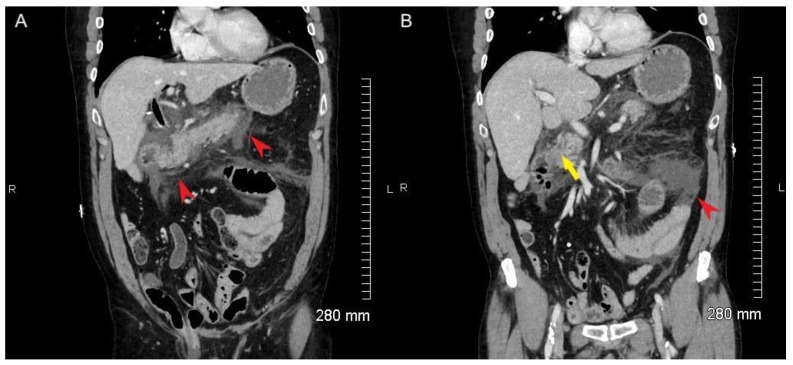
CT abdomen and pelvis with contrast demonstrates significant peripancreatic fluid and fat stranding extending to porta hepatis, consistent with acute pancreatitis (A,B, red arrowheads). The patient is status post cholecystectomy. There is pneumobilia, likely associated with prior cholecystectomy. The biliary tree is prominent with abnormal wall enhancement. The CBD measures 9 mm proximally and 5 mm distally. No definite signs of choledocholithiasis are seen in the distal CBD (B, yellow arrowhead).
